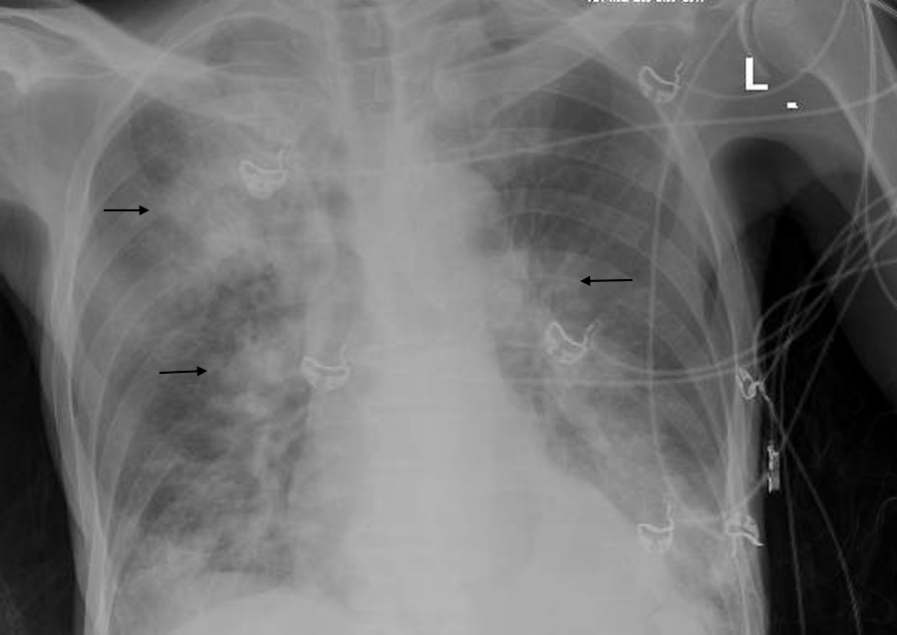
Chest radiograph showing diffuse opacification and emphysematous changes
